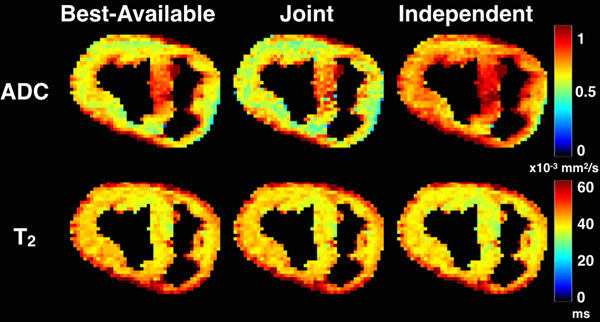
Ex vivo* ADC and T_2_ maps from 10 TEs and 6 DWI averages, respectively (left), 6 TEs and 3 DWI averages (right) and Joint reconstruction from 6 TEs and 3 DWI sets (center). Joint estimation maps were closer to the Best-Available T2 or ADC maps than the Independent T2 or ADC maps alone and came with no increase in scan time.
